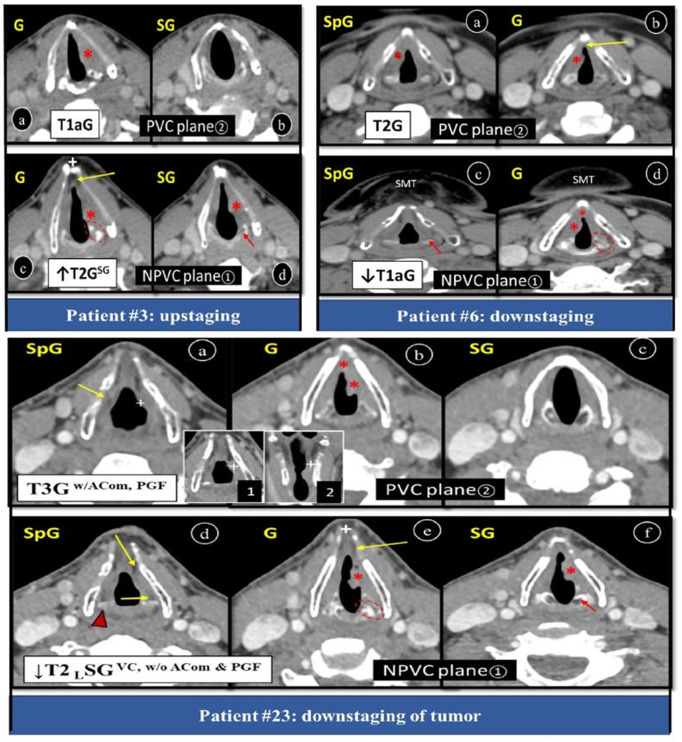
Axial post-contrast CT larynx showing change in T-staging of LC due to CT plane. Patient#3: Plane② (a, b) showing localised left glottic tumor, while plane① (c, d) showing glottic tumor extending to SG. Asterisks (*) showing areas of tumor extension (G, SG), dotted oval is CAJ at glottic level, short arrow is SG cricoid cartilage, long arrow is fat in ACom, (+) is thyroid notch, ↑ is upstage. Patient#6: Plane② (a, b) showing right glottic tumor extending to SpG, while plane① (c, d) showing localised right glottic tumor with ACom extension only. Asterisks (*) showing areas of tumor extension (G, ACom, SG), dotted oval is CAJ at glottic level, short arrow is SpG AC, ↓ is downstage. Patient#23: Showing change in T-staging, location, PGF and ACom involvement due to CT plane. Plane② (a, b) showing left glottic tumor extending to SpG with minor PGF involvement, while plane① (c, d) showing bulk in SG with G extension and normal PGF. Asterisks (*) showing areas of tumor extension (PGF, G, SG), dotted oval is CAJ at glottic level, arrowhead id upper part of AC in SpG, red arrow is SG cricoid cartilage, all yellow arrows are fat, ↓ is downstage, (+) is thyroid notch. Note false anatomical landmarks of G in NPVC plane, i.e., fat, SMT and open thyroid notch. *G = glottis, SpG = supraglottis, SG = subglottis, ACom = anterior commissure, NPVC = non-parallel vocal cord, PVC = parallel vocal cord, AC = arytenoid cartilage, CAJ = cricoarytenoid joint, SMT = submental triangle, ACom = anterior commisure, PGF = paraglottic fat.*
